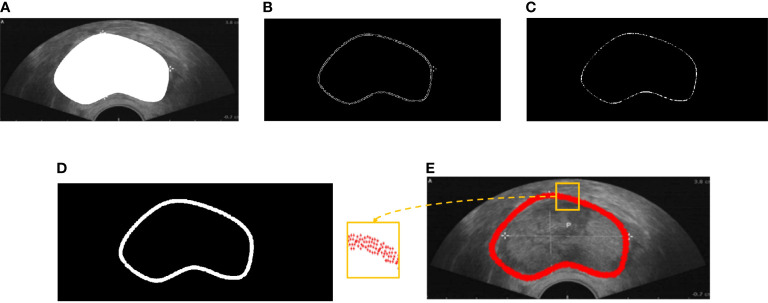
(A) Semantic segmentation of prostate ultrasound image. (B) Edge detection of the prostate. (C) Prostate boundary after erosion. (D) Erode prostate boundary after dilation. (E) Prostate contour.
